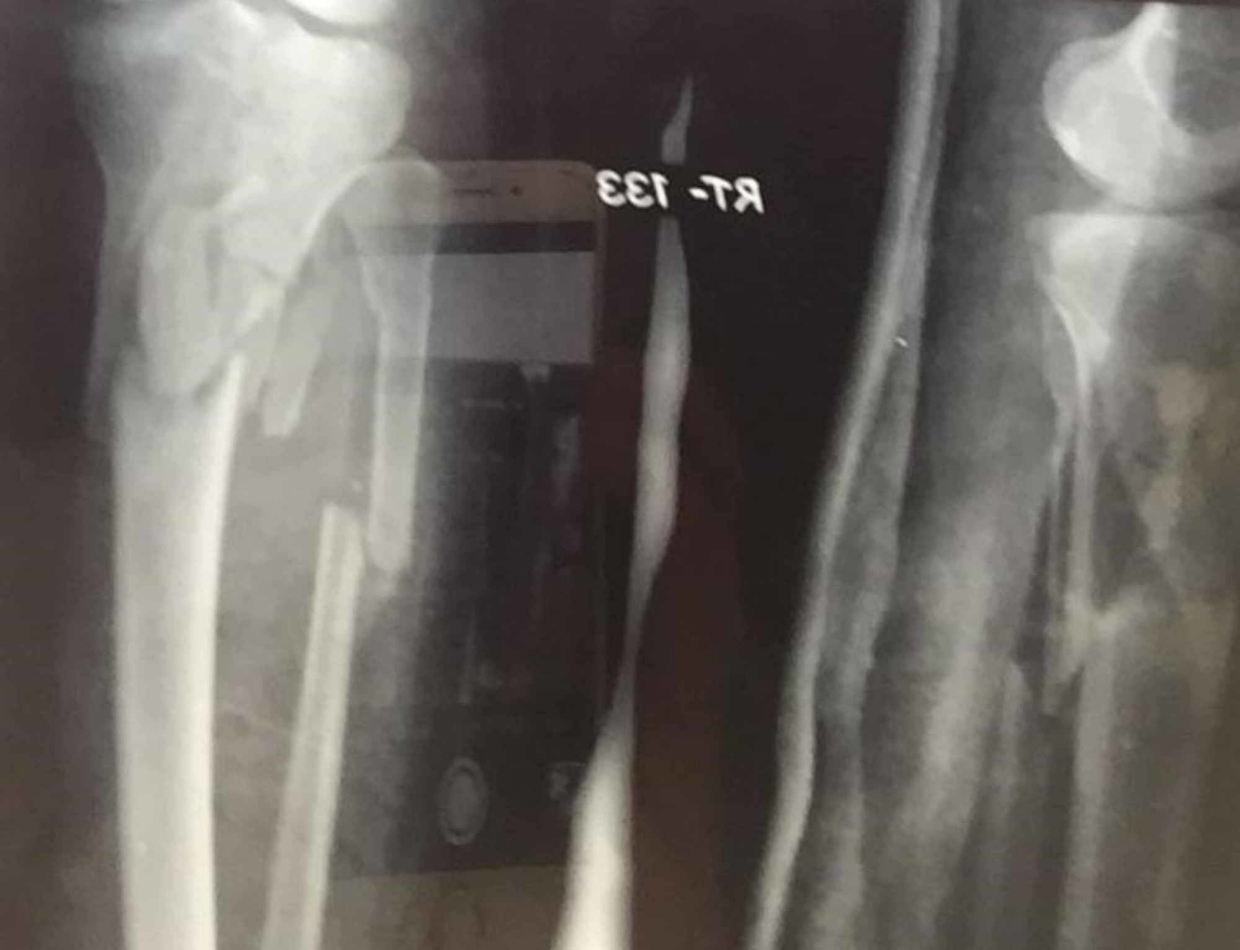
Preoperative plain radiograph of one patient showing a comminuted tibial plateau fracture, Shatker's type VI, along with a fracture of the proximal part of the fibula
